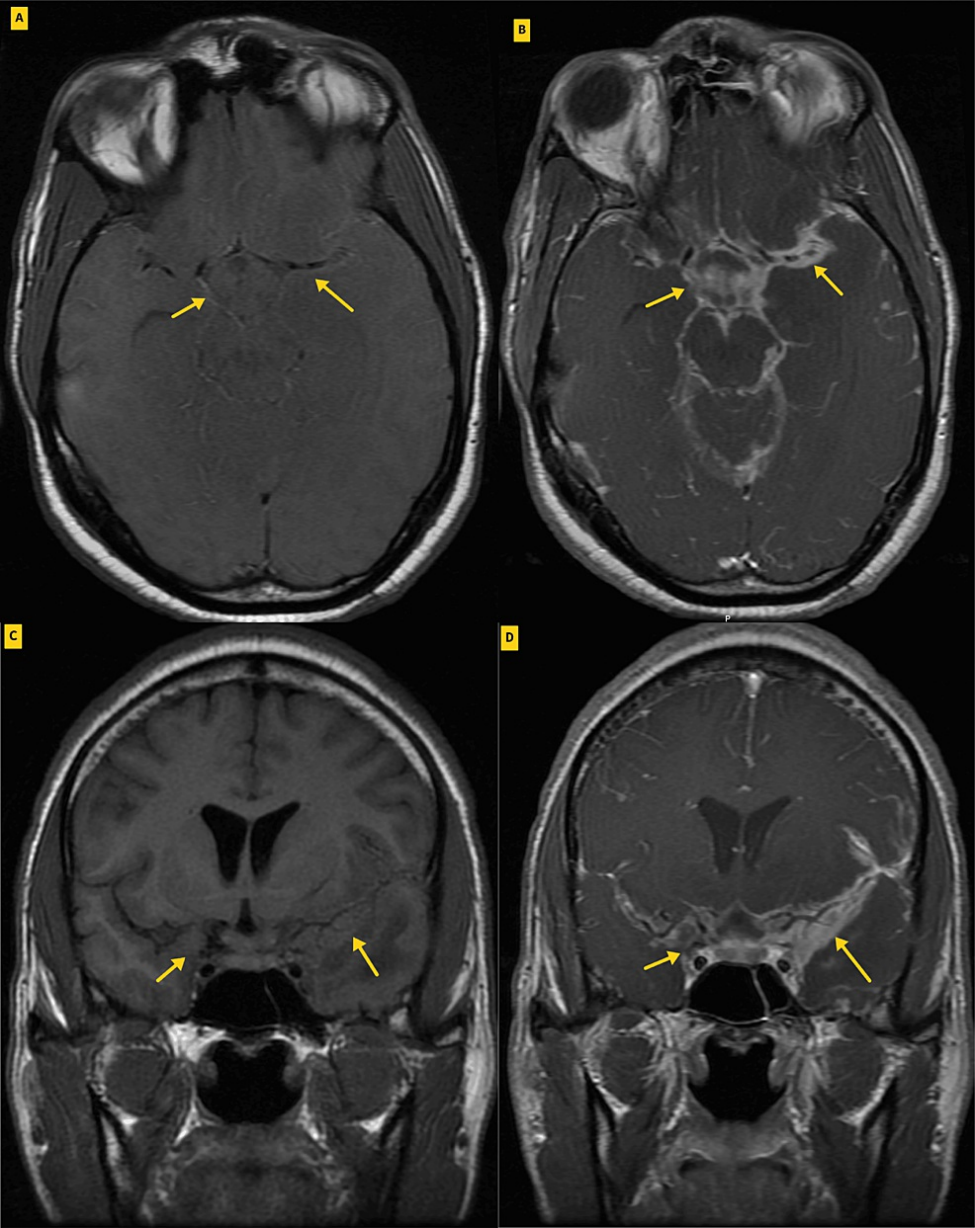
Representative axial (A and B) and coronal (C and D) pre-contrast (A and C) and post-gadolinium (B and D) magnetic resonance imaging (MRI) through the suprasellar cistern demonstrate diffuse leptomeningeal enhancement consistent with meningitis.
